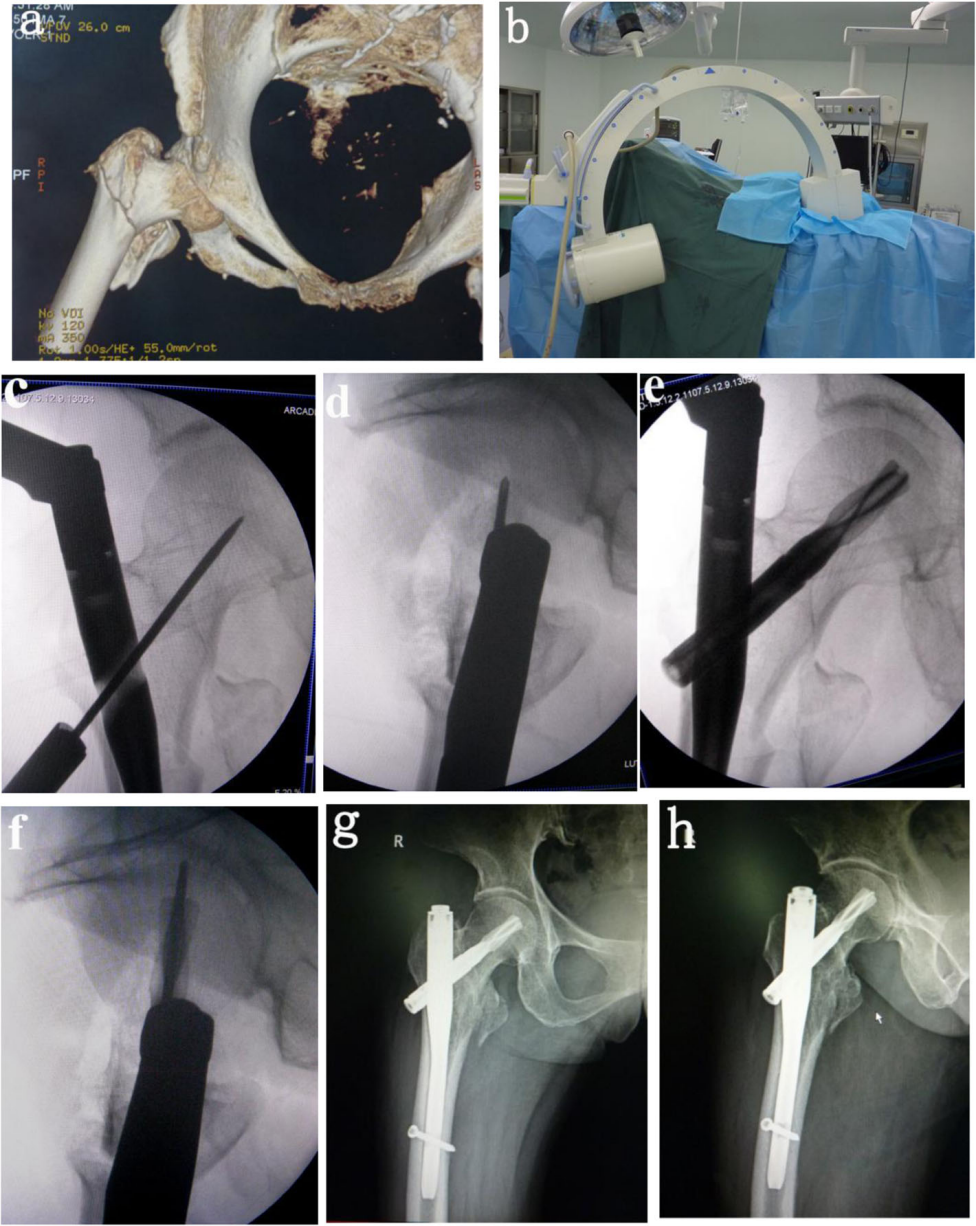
Female, 72 years old, right femoral intertrochanteric fracture caused by road traffic injury (AO A2.1). Surgical treatment was performed 3 days after admission. A three-point positioning method was used to implant femoral neck screws. a Preoperative three-dimensional CT examination showed a right intertrochanteric fracture and a lesser trochanter fracture with displacement. b During the operation, the fluoroscope was tilted 15° to show the lateral image of the femoral neck. c Intraoperative anteroposterior fluoroscopy 3-point positioning to ensure that the guiding needle was in the middle of the femoral neck. d During the operation, using lateral fluoroscopy, the 3 points were located to ensure that the guiding needle was in the middle of the femoral neck. e Perspective positive position after placing the spiral blade. f Perspective on the posterior lateral position when inserting the spiral blade. g, h The X-rays were followed up 2 months after surgery
